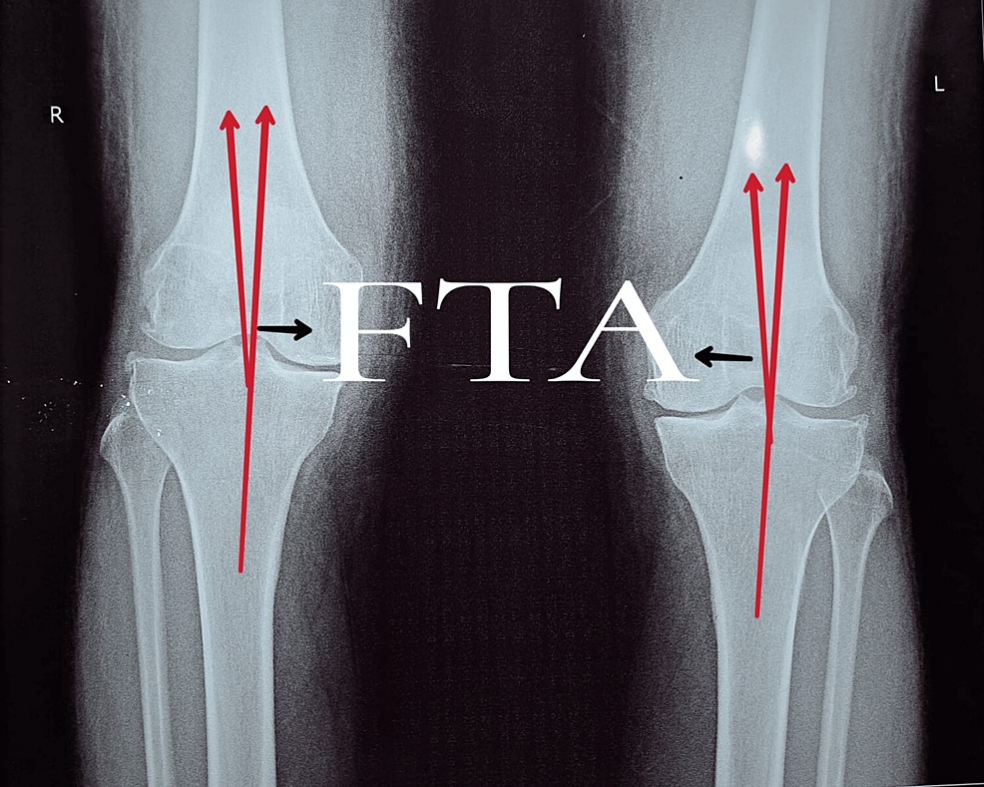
Medial joint space narrowing (left > right) in X-ray of bilateral knee joint in anteroposterior view. Femoral-tibial angle (FTA) for the right and left knee joints is depicted in the X-ray.
